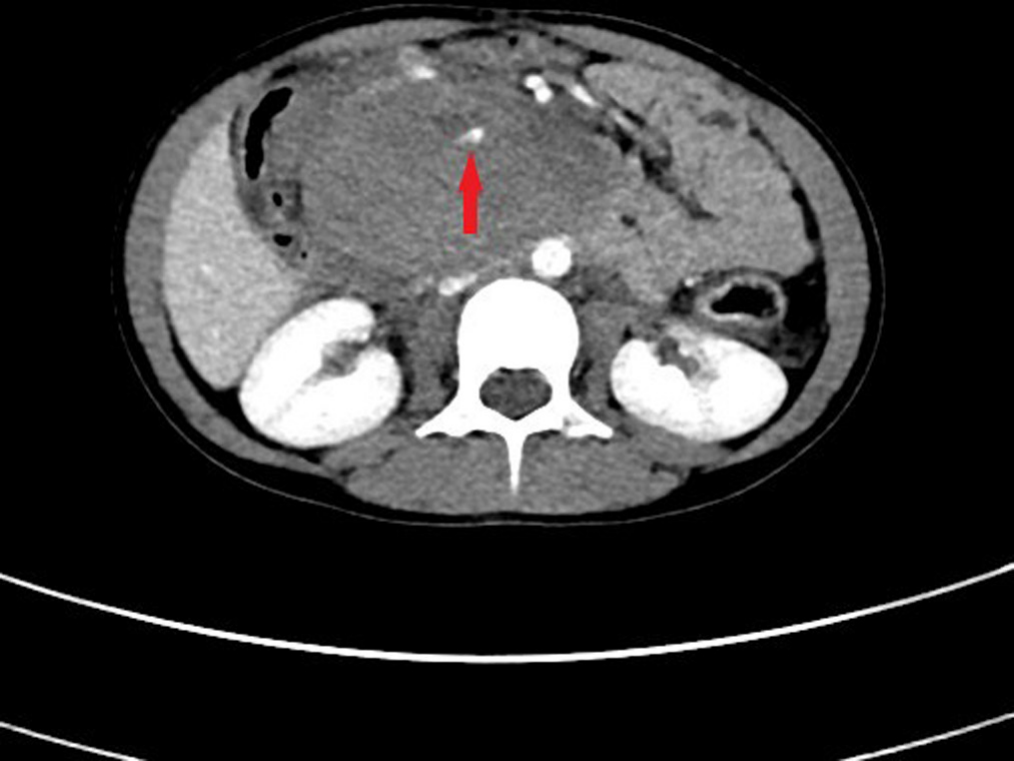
Axial CT image of abdomen demonstrates a large intramural hematoma in second and third part of duodenum with focal contrast extravasation (arrow) consistent with active bleeding.
